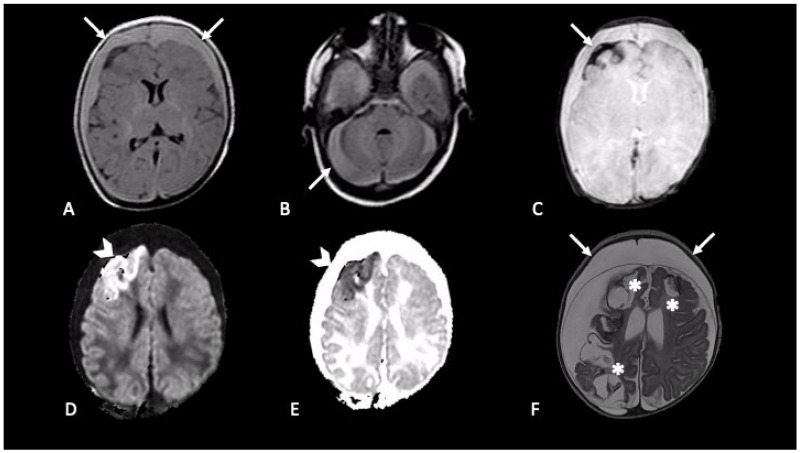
Newborn first accessed in the emergency department with fever and vomit, then was hospitalized four days later due to generalized seizures. CT showed mixed-density bilateral fluid collections (not shown). MRI demonstrated bilateral subdural CSF-blood collection, supra- and infra-tentorial (A,B axial FLAIR, arrows); subarachnoid blood products are revealed by susceptibility weighted imaging in right frontal lobe (C, axial SWI, arrow); intraparenchymal cortical-subcortical hemorrhage in the right frontal lobe (D,E, axial DWI and ADC map, arrows-head). One-month follow-up MRI (F, axial T2) showed malacic evolution of intraparenchymal damage (asterisks) and bilateral subdural hygromas (arrows).
